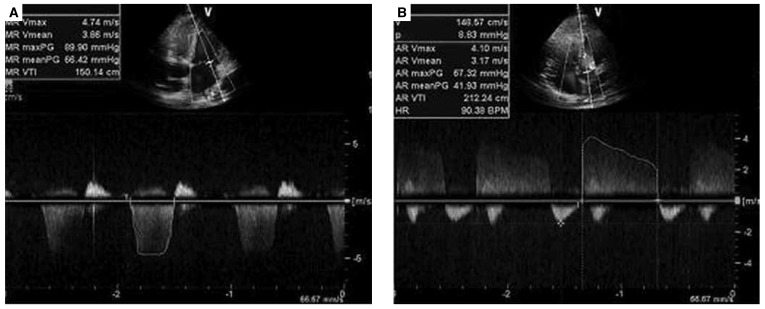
Mitral valve regurgitation in apical four-chamber view (A), and aortic regurgitation in apical five-chamber view (B) with pulse-wave Doppler.
